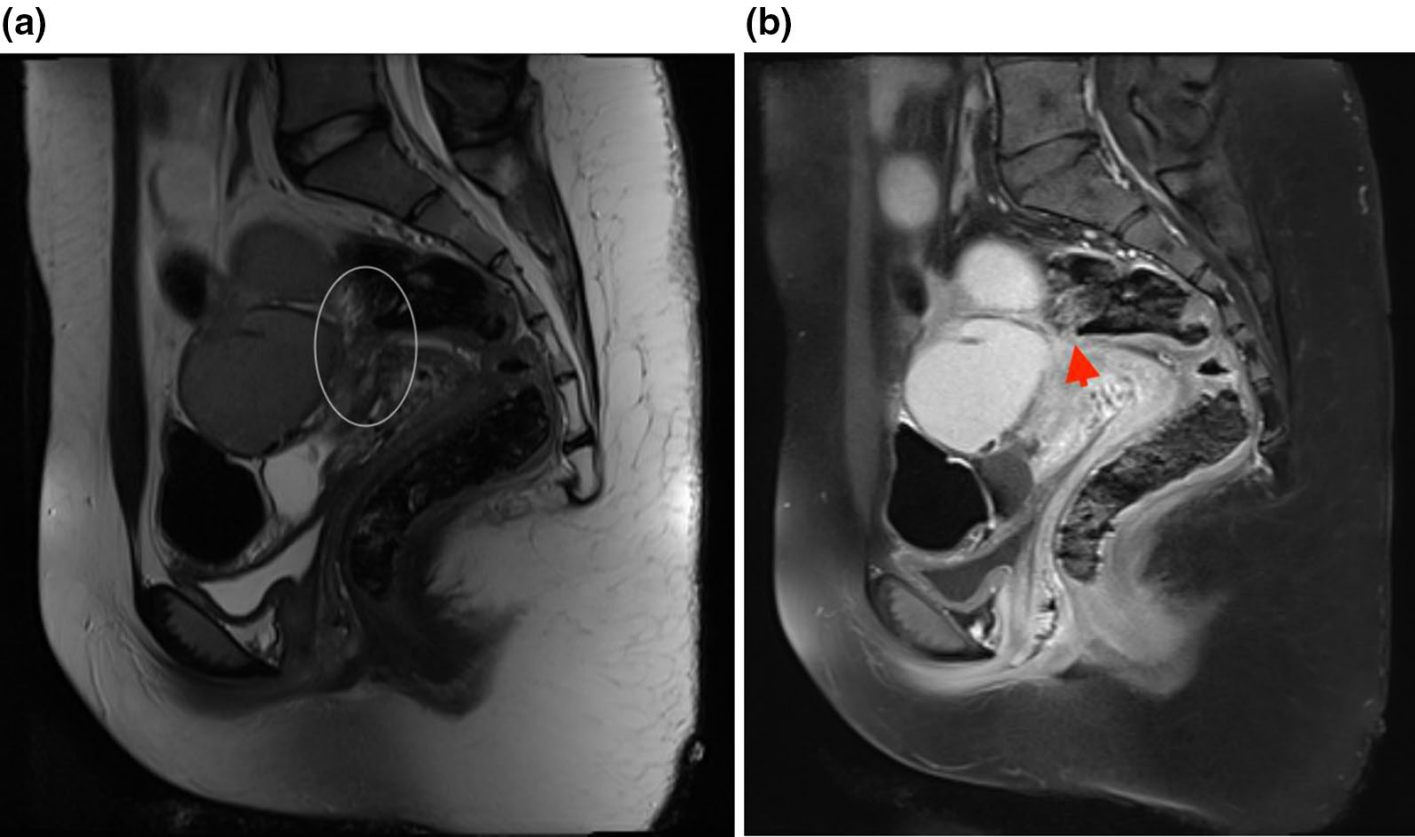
a T2 weighted sagittal MRI image showed involvement of posterior cul-de-sac region as a site of DIE with some adhesions with rectal wall and possibility of DIE nodule at the rectum. b T1 weighted sagittal fat-suppressed image with contrast in the same patient showed delineation of the rectal wall (arrowhead) with some tenting due to adhesion but no definite rectal implants
